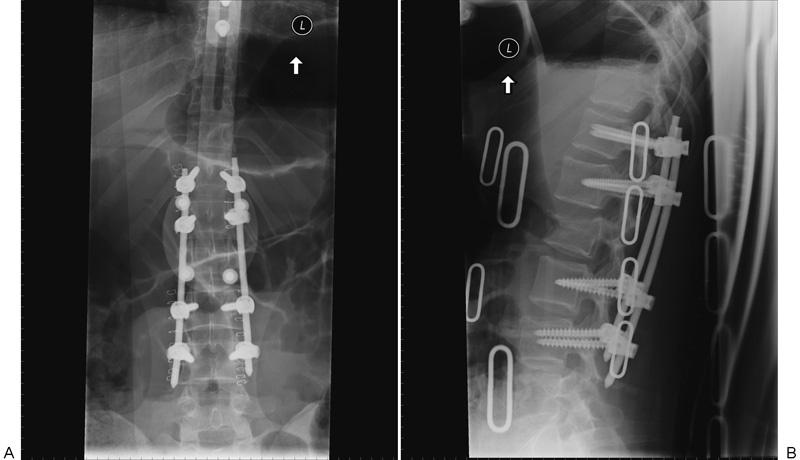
Postoperative plain film assessment of screw placement. Excellent pedicle screw placement is seen. (A) Anteroposterior view. (B) Lateral view.
